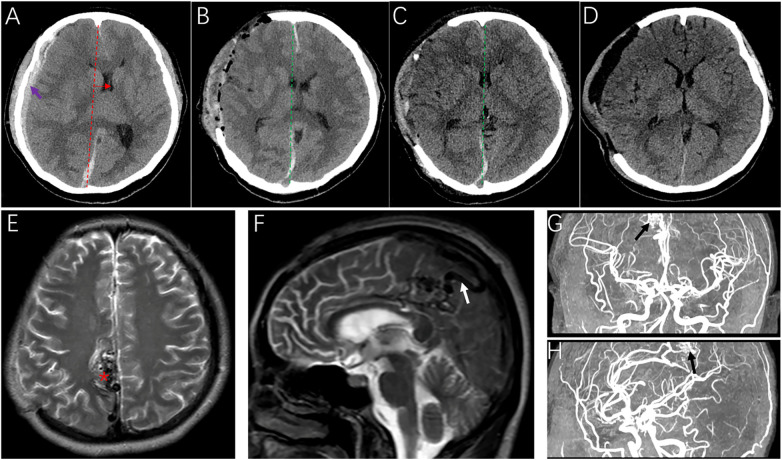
Radiologic evolution demonstrating the staged “damage control” management of a ruptured AVM in pregnancy. (A) Preoperative noncontrast computed tomography (CT) revealing a massive right-sided acute subdural hematoma (ASDH) with severe mass effect (purple arrow), effacement of the right lateral ventricle, and significant midline shift (the red dashed line and the red horizontal arrow), indicating impending uncal herniation. (B,C) Early postoperative CT scans showing the evacuation of the hematoma and the progressive restoration of the midline (green dashed lines) following emergent right decompressive craniectomy (D) Postoperative day 14 CT showing resolution of the brain swelling without midline shift. (E,F) Postpartum magnetic resonance imaging (MRI) (T2-weighted sequences) demonstrating an abnormal vascular signal (red asterisk) in the right frontoparietal lobe with a prominently dilated cortical draining vein (white arrow). (G,H) MRA confirming the presence of the arteriovenous malformation nidus (black arrows).
